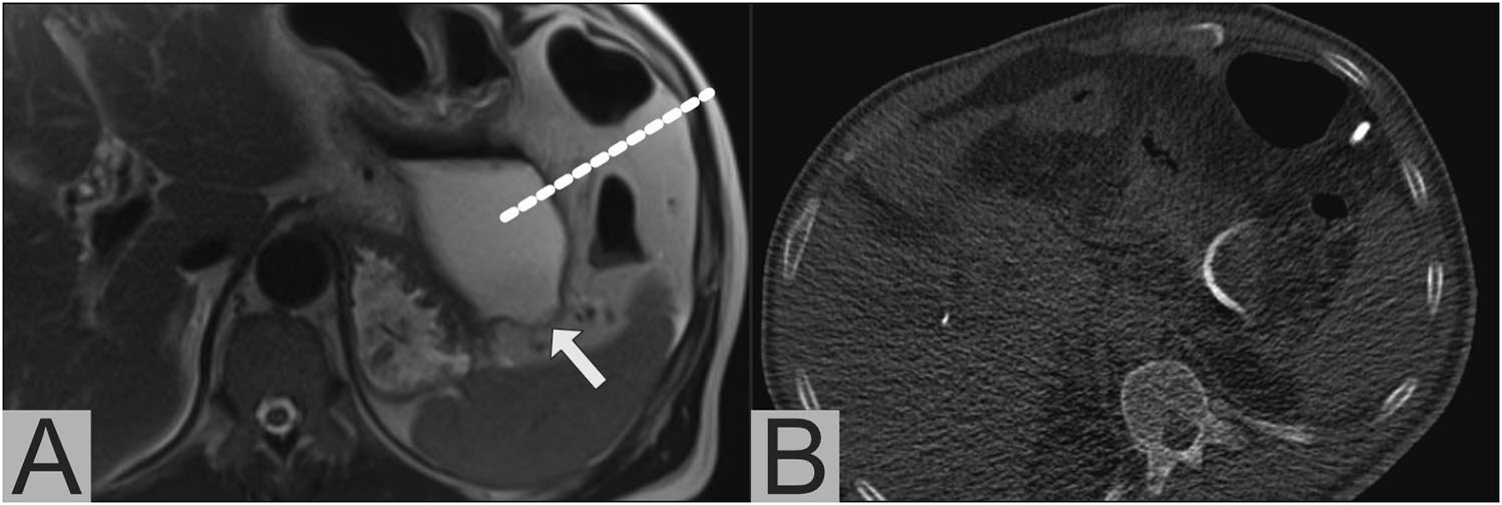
PoSSe MRI showing a perigastric collection (arrow) after pancreaticoduodenectomy, planning of CT-guided drainage insertion is marked by the dotted line (a); CT-Fluoroscopy showing the successful inserted drainage via the planned puncture tract (b)
